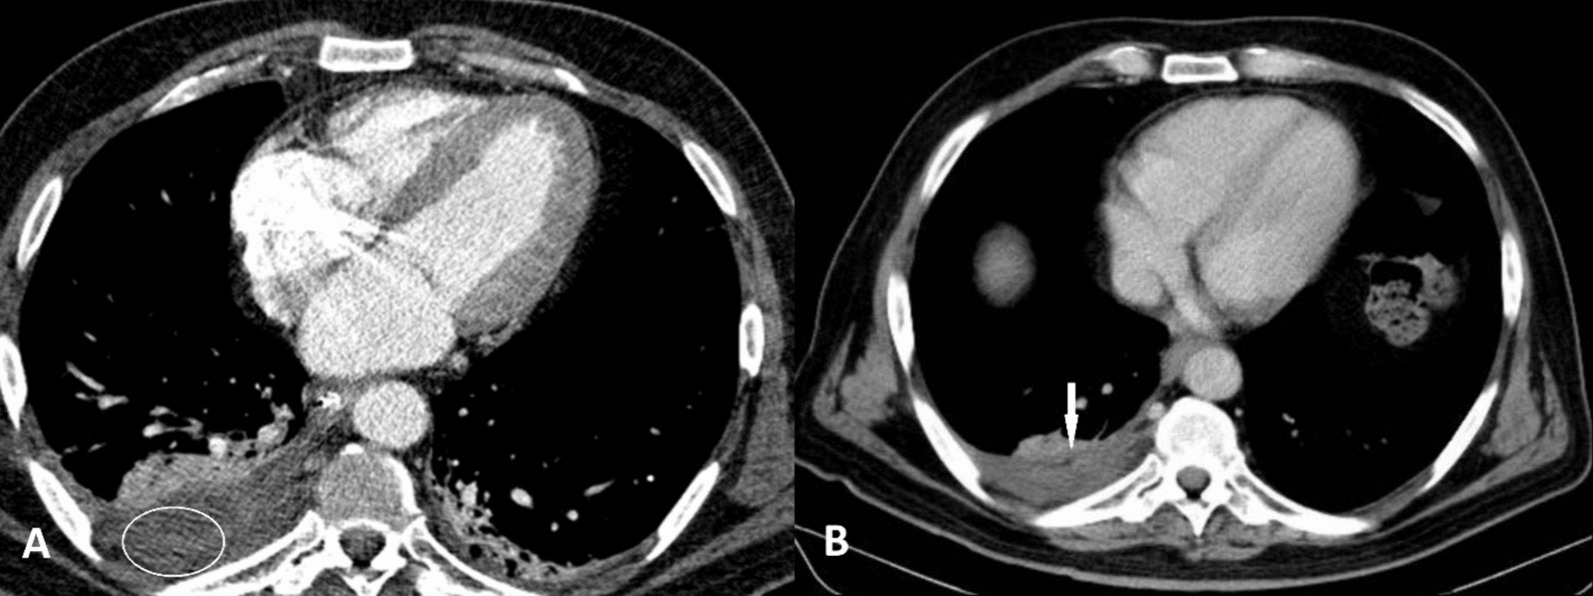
Chest computed tomography scan with IV contrast. A at the time of admission, showed a loculated fluid collection with mild wall enhancement in the right side posterior pleural space in favor of the pulmonary abscess (white oval). B After treatment, a gradual decrease in the size and extent of the abscesses was observed (arrow)
